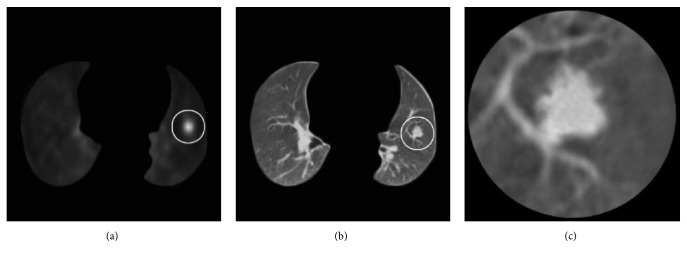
ROIs extraction of the pulmonary nodule. (a) Location of the nodule in PET image; (b) location of the nodule in CT image corresponding to PET image (a); (c) ROI of the pulmonary nodule.
